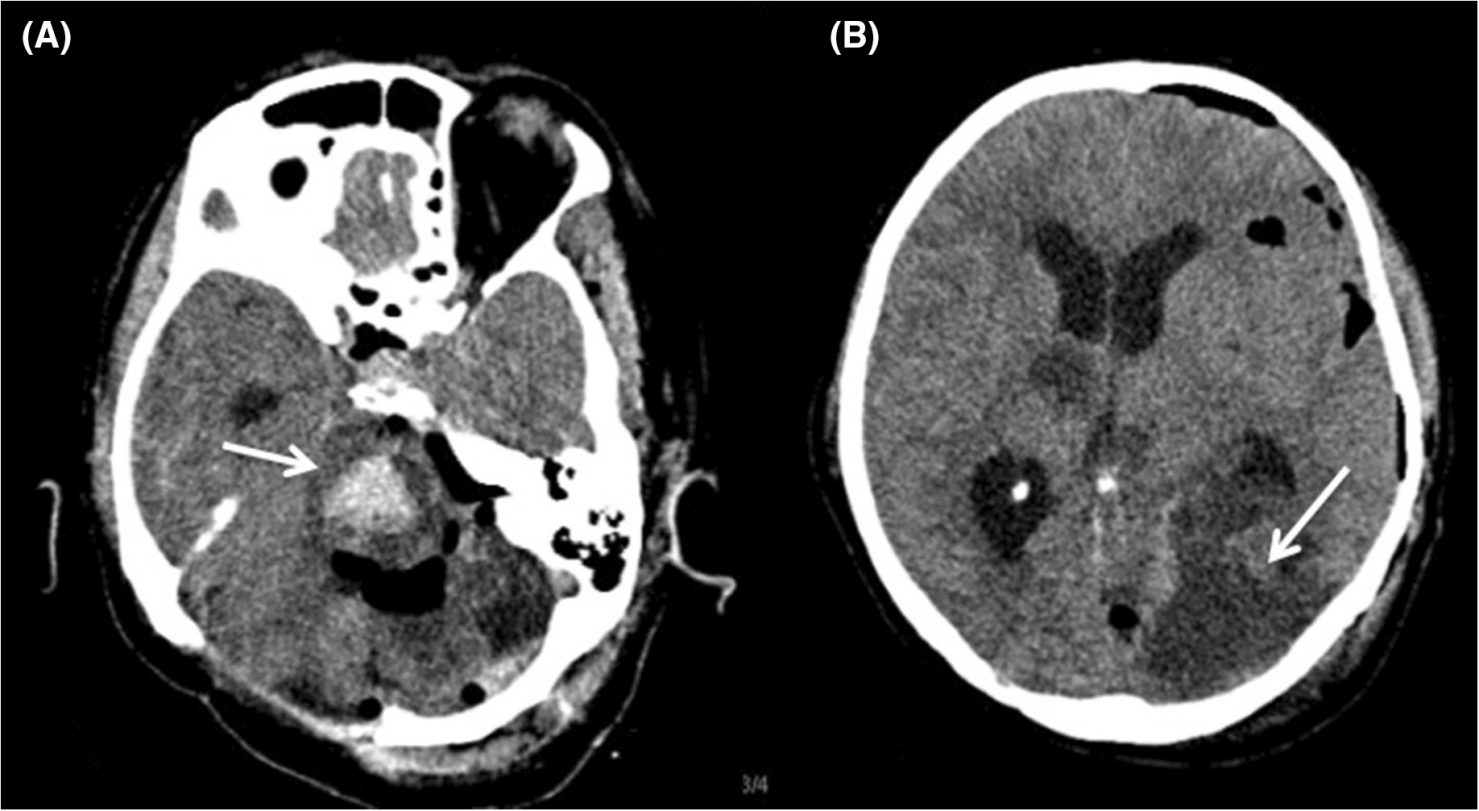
Skull computed tomography performed after epidural hematoma evacuation. (A) Brainstem hematoma. (B) Occipital ischemia suggesting brain herniation.
